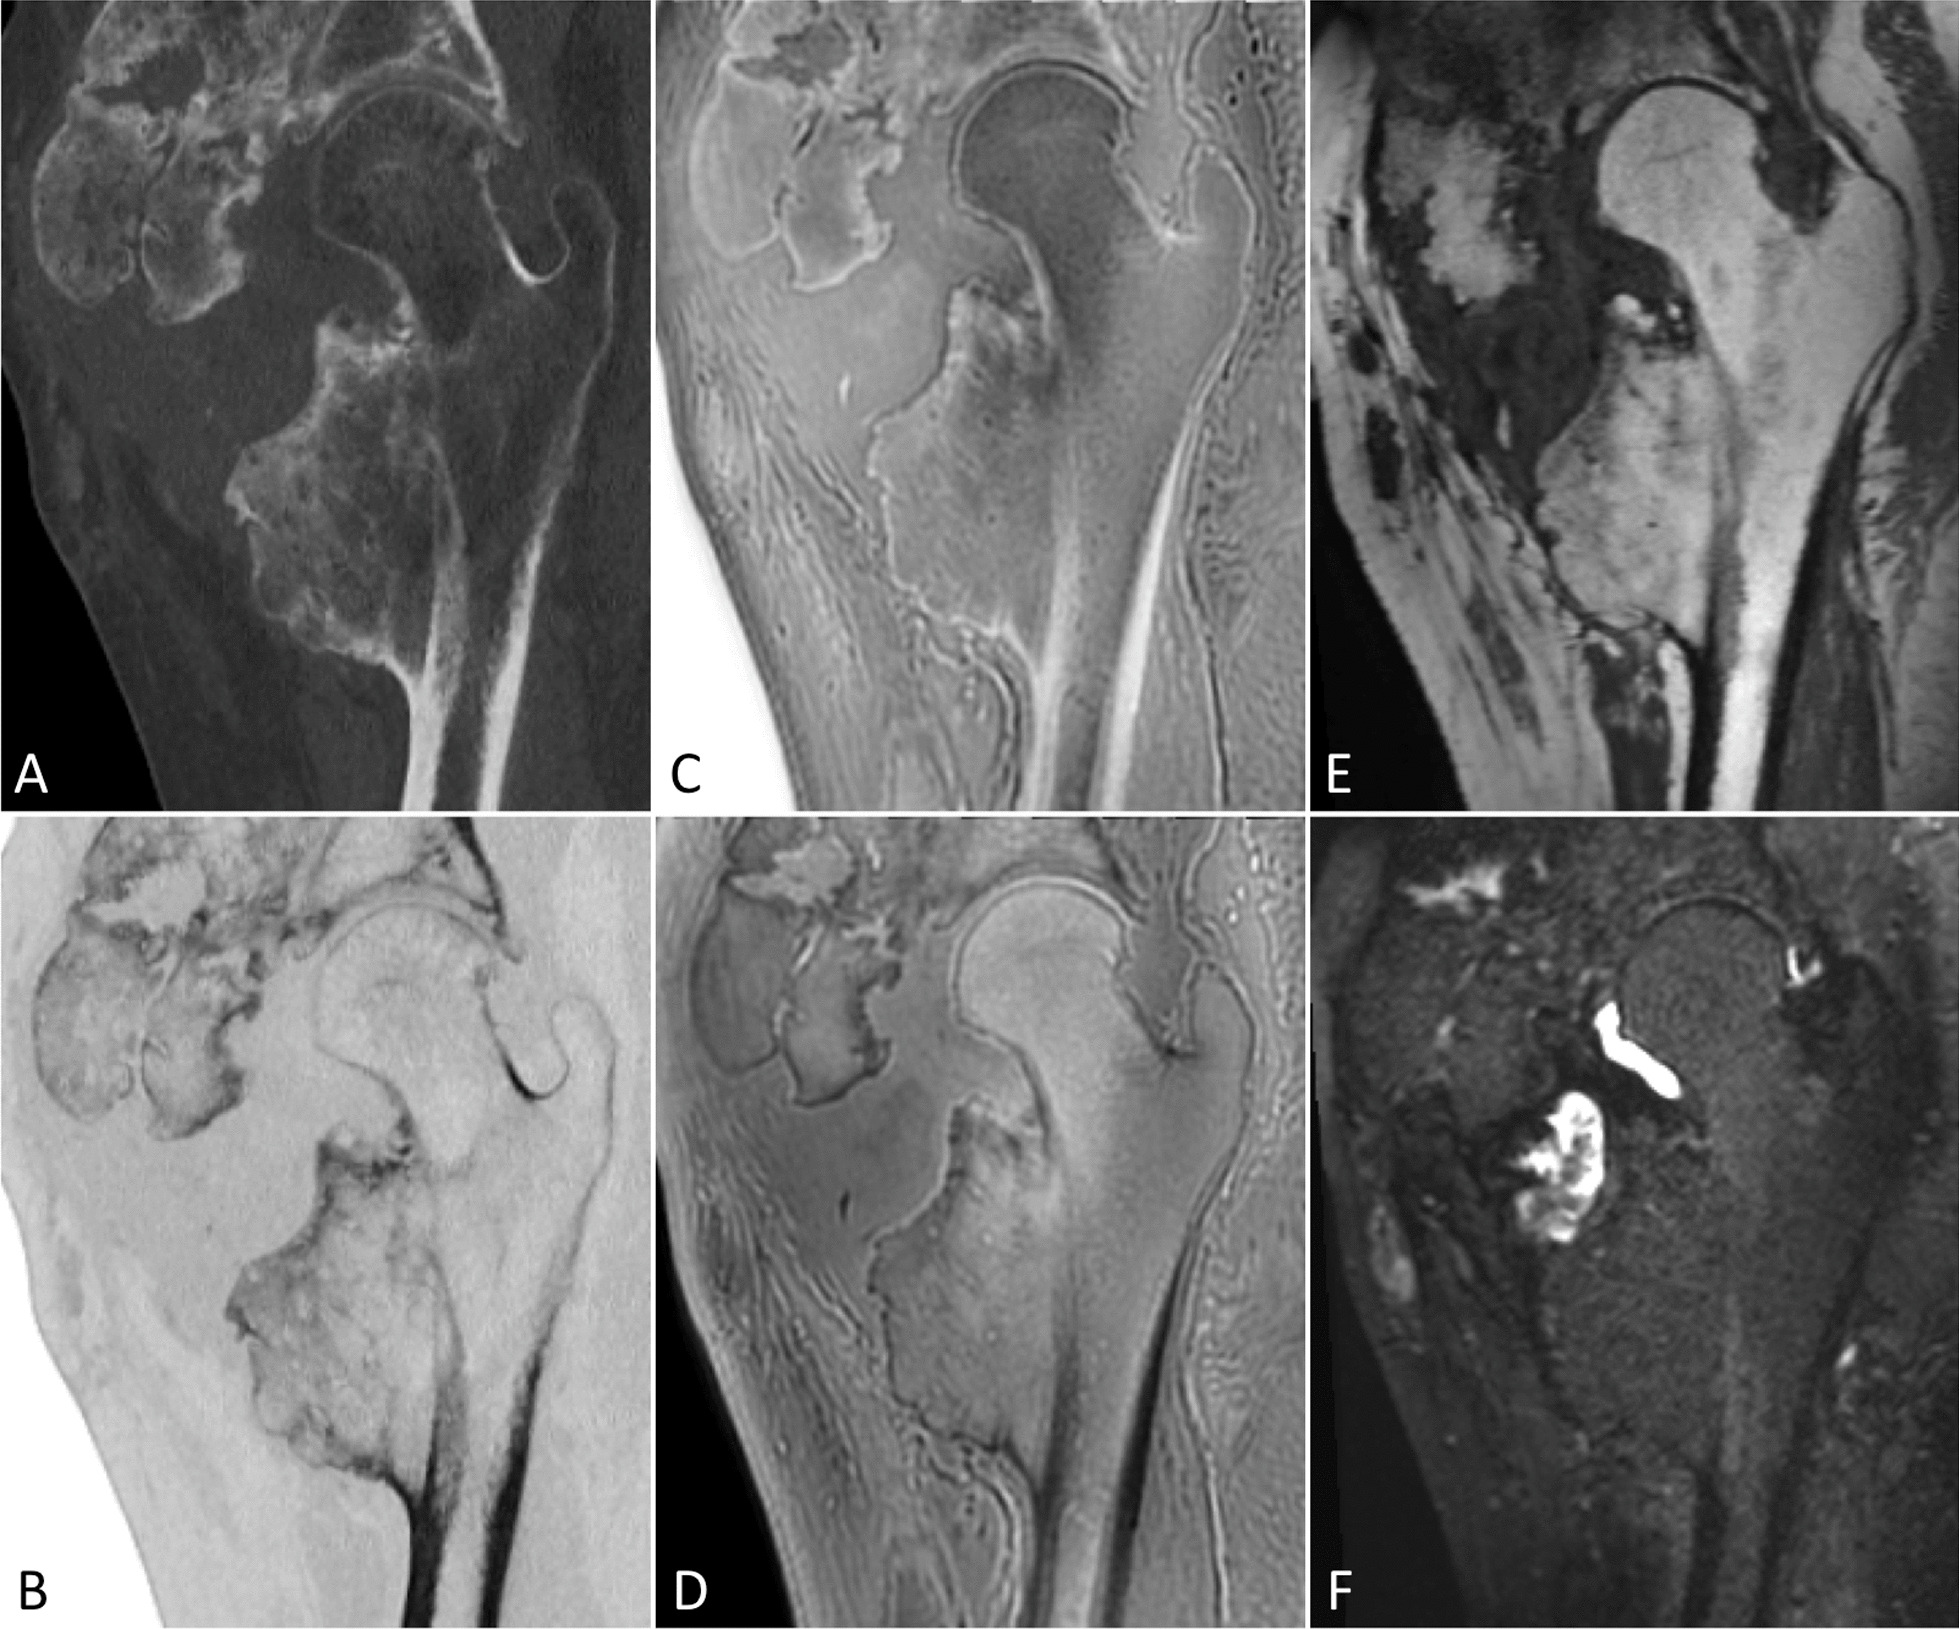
Heterogeneous multifragmented NHO of the right hip with pseudarthrosis. Comparative sagittal images in gray scale (A) and inverted gray scale (B) on CT, ZTE sequence with inverted gray scale (C), ZTE sequence (D), T1-weighted (E) and STIR sequences (F) on MRI. Note the articular effusion in the pseudarthrosis visible on the STIR sequence
